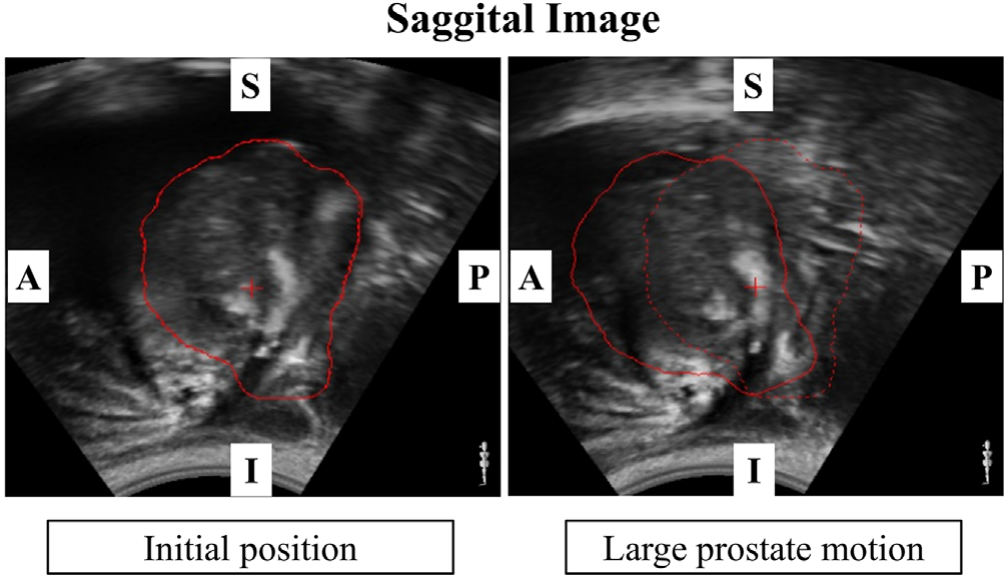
Example of an ultrasound image with one of the large prostate motions for volumetric modulated arc therapy (VMAT). Prostate suddenly moved in the AP direction owing to a rectal gas movement
